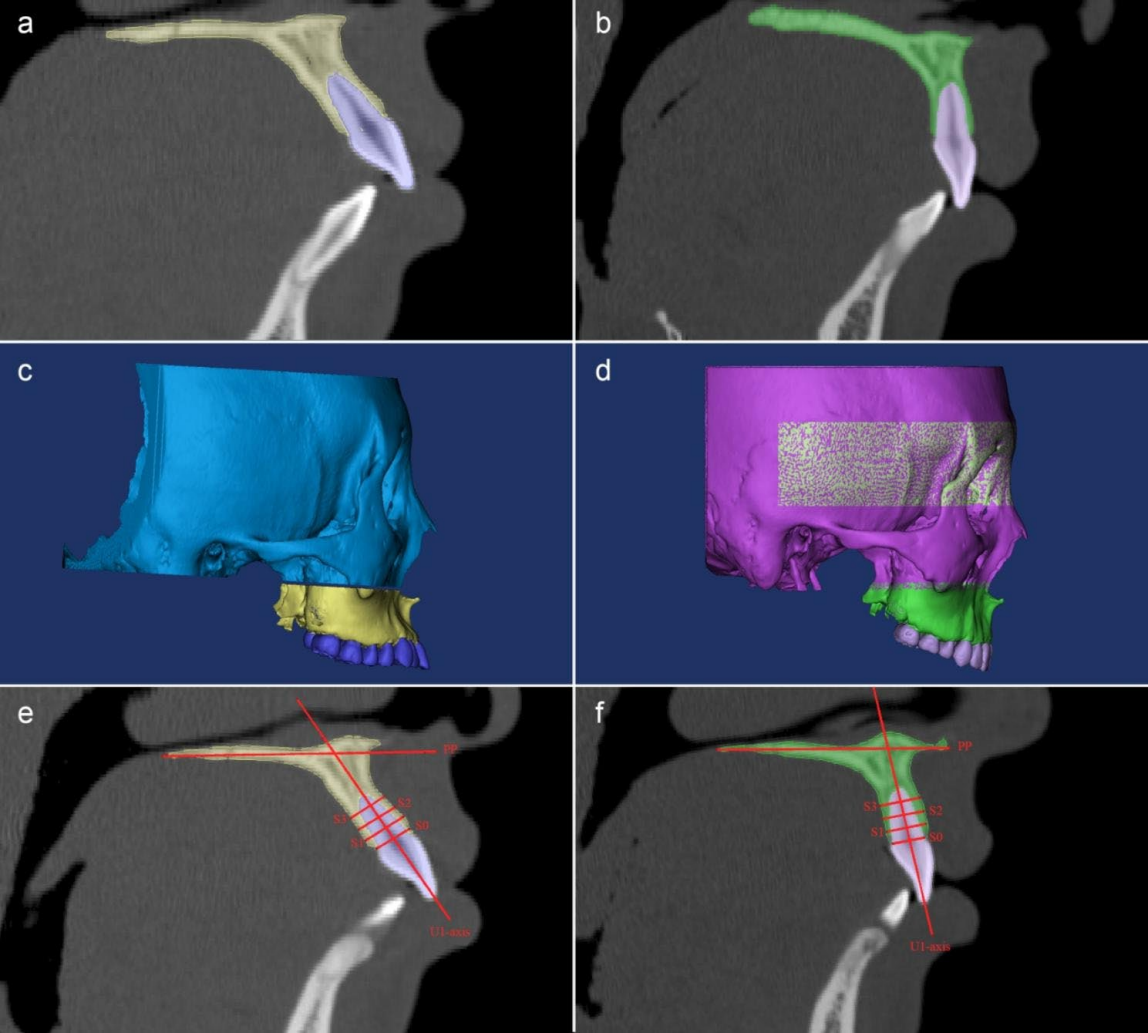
The 3D segmentation and reconstruction of T1 and T2 CBCT images. The maxillary teeth, maxillary alveolar bone and cranial base of T1 (a, c) and T2 (b, d) were reconstructed with various threshold values. In the U1-axis sagittal plane, the labial, palatal and total alveolar bone thicknesses were measured in three slices separated by 3 mm (S1, S2 and S3, respectively). We also assessed the U1-PP angles of T1 (e) and T2 (f)
